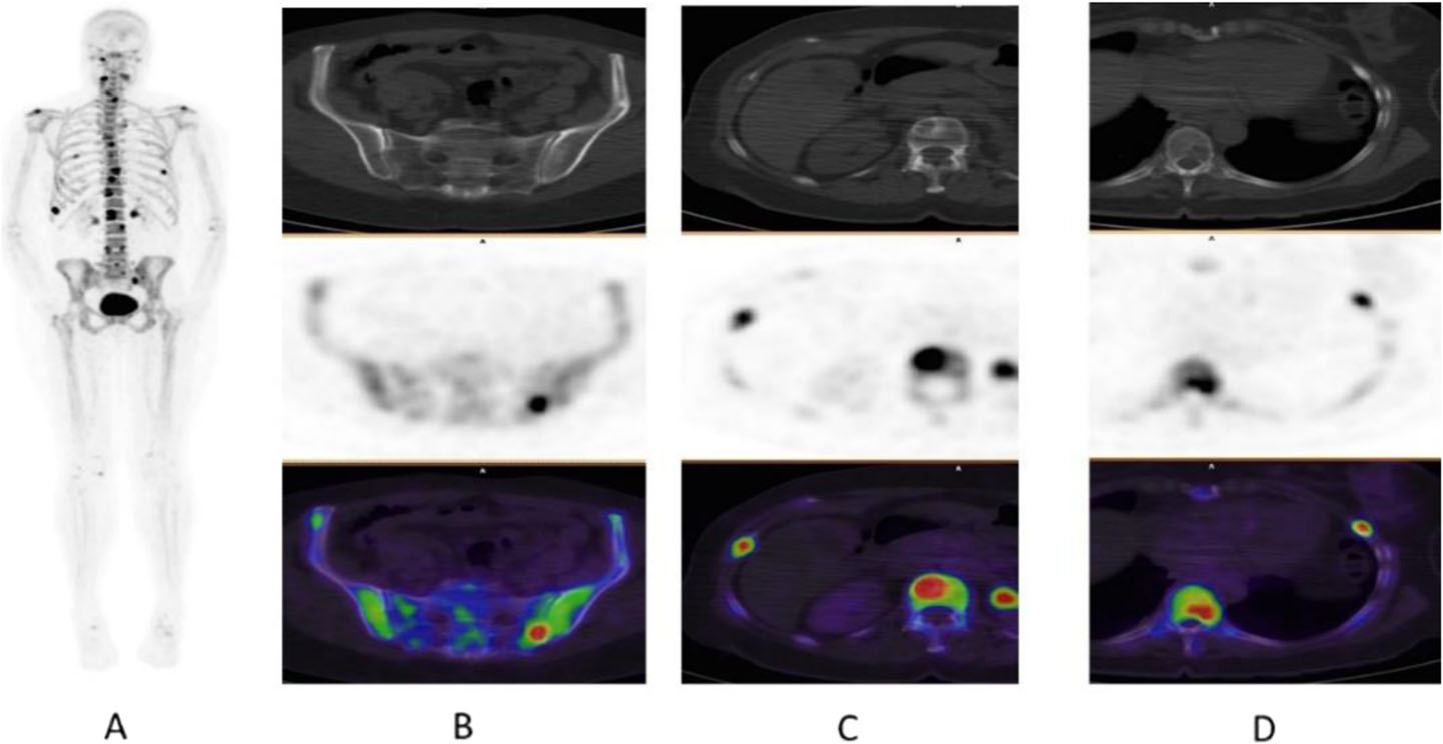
^18^F-NaF, breast cancer staging. *Clinical history*: 55 y.o. woman recently diagnosed with breast cancer (ductal adenocarcinoma, ER+, PR, and HER2 neg), asymptomatic. *PET/CT findings*: multiple foci of increased tracer uptake consistent with skeletal metastatic spread (a MIP). Note the heterogeneity of the CT appearance in this patient: some lesions are osteolytic (b), others are sclerotic (c) or do not show any CT anomaly (d)
